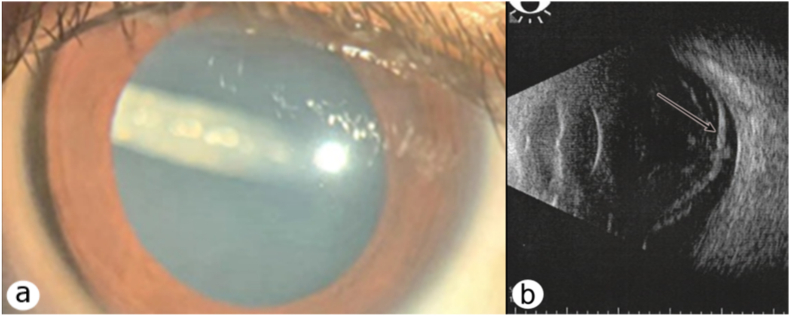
Case №3. a. On slit-lamp examination, a larva is visualized in the vitreous posteriorly to the lens capsule. b. Ocular ultrasound demonstrating retinal detachment (white-black arrow).
